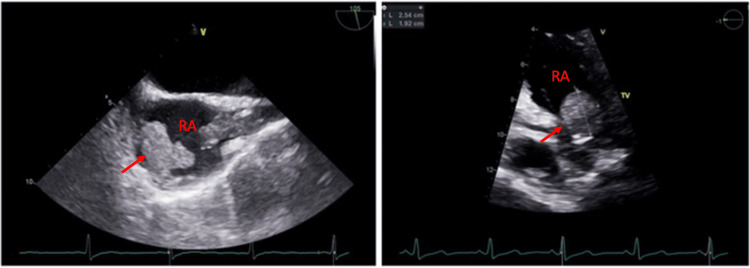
Transesophageal echocardiography showing the right atrial thrombus (red arrows).
